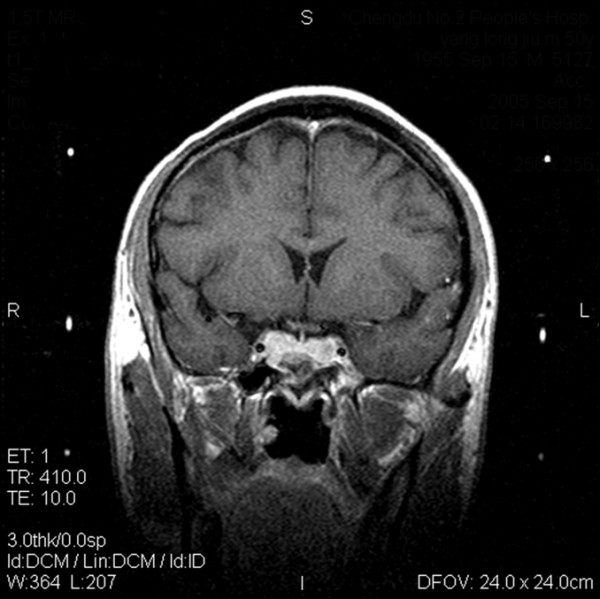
Typical MRI scan changes in ACTH adenoma. Coronal T1-weighted postcontrast MRI scan at left and right, obtained in Patient 1, a 30-year-old man who presented with ACTH adenomas and consistent headache 2 years before undergoing GKRS. An enhancing mass lesion is seen in the sella turcia with extension to bilateral internal carotid artery. Patient 1's serum ACTH level was 381.6 pg/ml, and his blood pressure was over 180/120 mmHg. The patient was treated with MASEP GKRS, and MRI was performed for treatment planning. 26 Gy defined to the 50% isodose line is used to cover the full extent of the pituitary tumor in all three planes.
